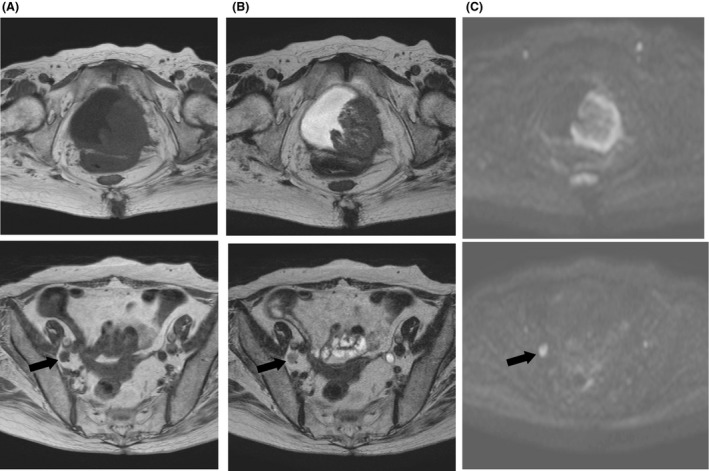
Magnetic resonance imaging. A huge mass (60 × 55 mm) in the bladder with lymph node enlargement (arrow) in the internal iliac region was observed. (A) T1‐weighted; (B) T2‐weighted (C) diffusion‐weighted.
